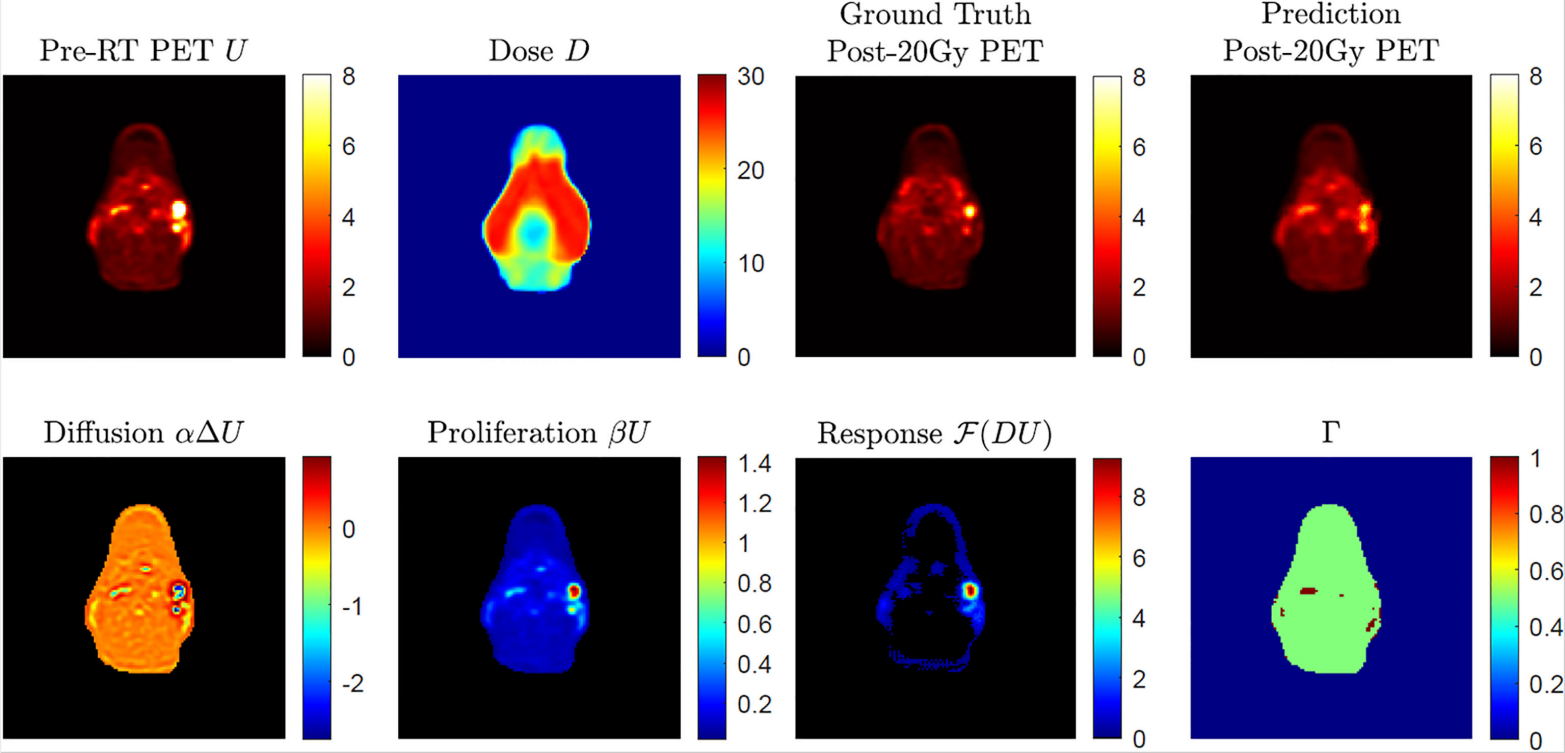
An example of post-radiotherapy ^18^FDG-PET image outcome with given pre-radiation ^18^FDG-PET image and dose distribution map D, with a breakdown of predicted biological effects (diffusion, proliferation, and dose response in absolute value) in Eq. (2). The 2D Gamma (Γ) test result was obtained through acceptance criteria of 5%/10 mm.
